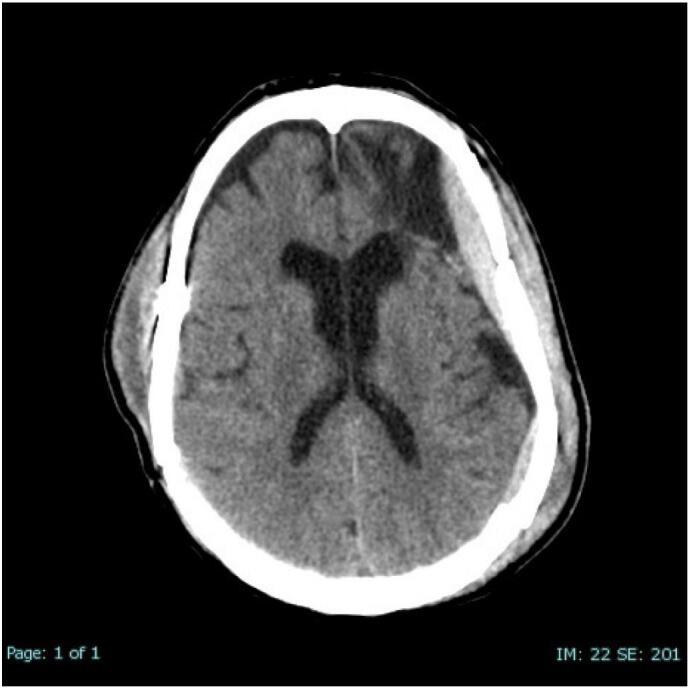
Follow-up CT scan - Demonstrating spontaneous resolution of bilateral subdural hematomas.
